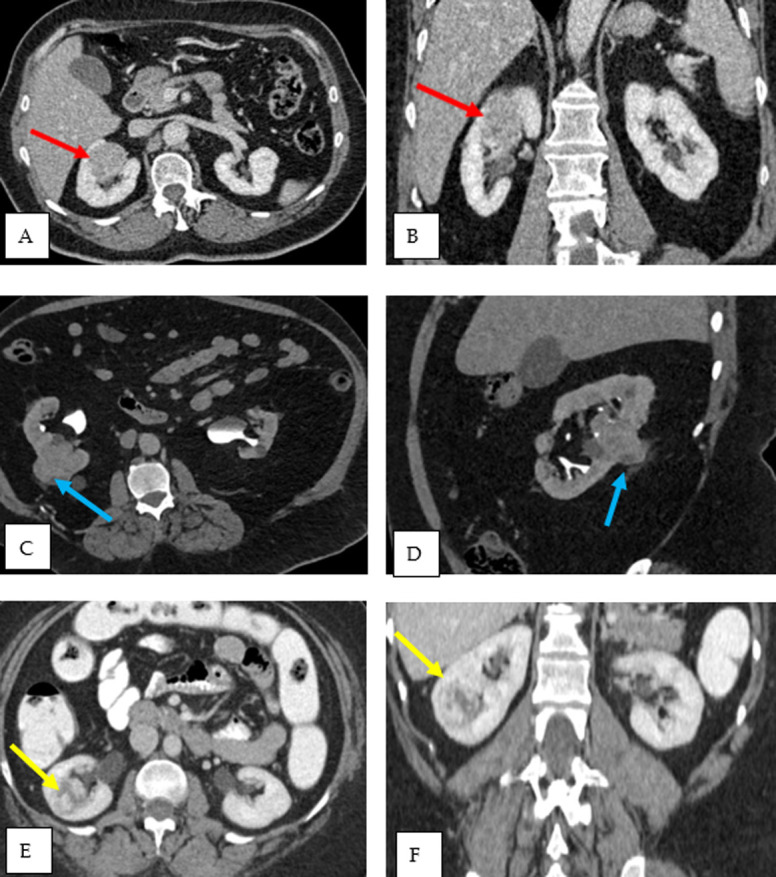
renal masses of three patients by contrasted CT scan; patient 1 (A, B)
